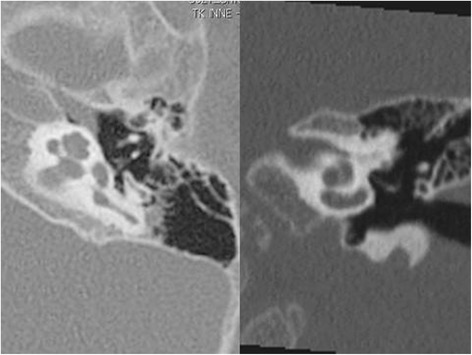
HRCT image (axial-left and coronal-right) of the left temporal bone before the first CI surgery at 1 year of age showing no middle ear pathology
